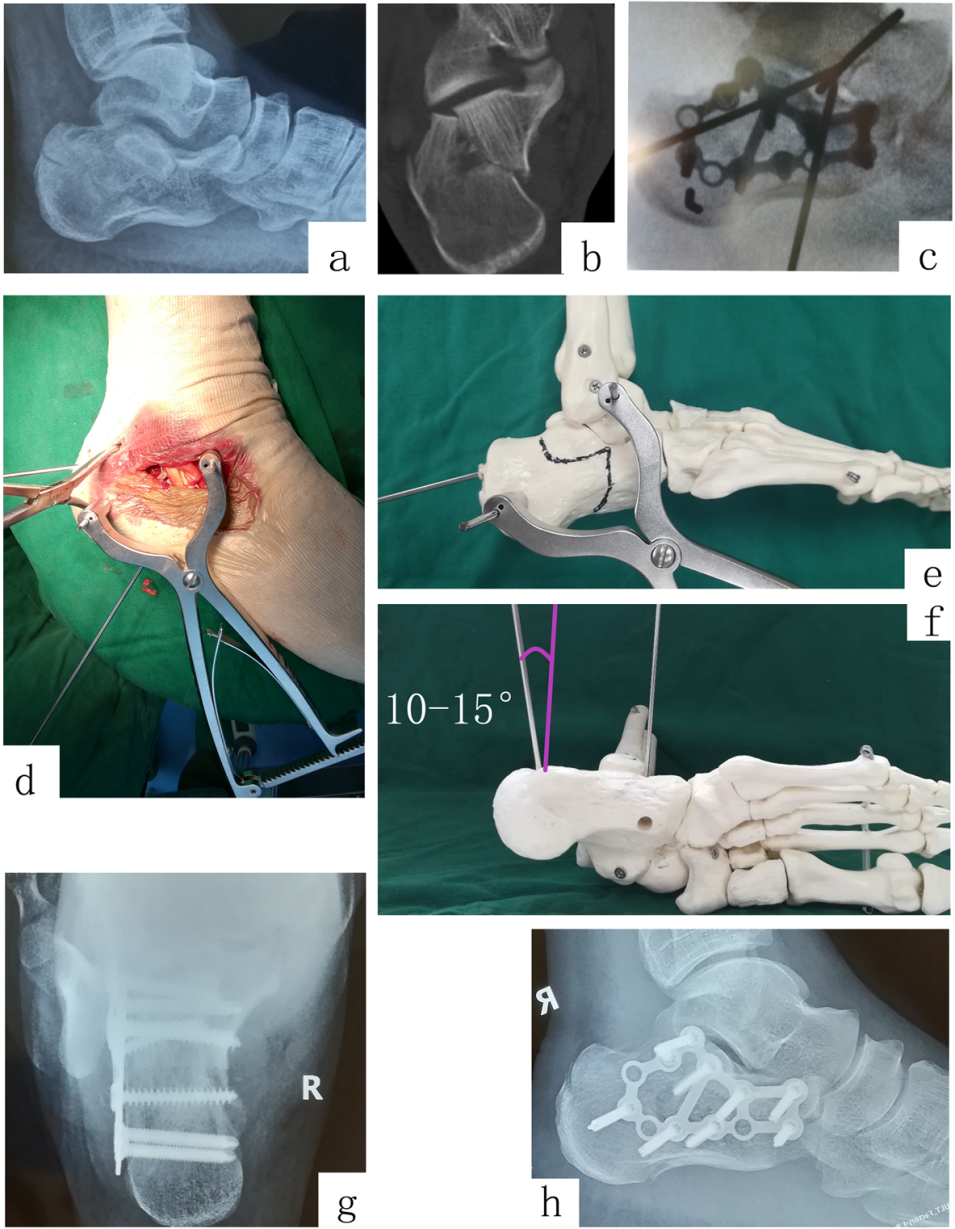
A 27-year-old male patient falls from a height of 2 m. Preoperative lateral (a) and axial (b) radiograph showed left calcaneus fracture with reduced Böhler angle (3°), length (80.1 mm), height (74.7 mm), and width (48.7 mm). The preoperative horizontal computed tomography image showed Sanders type IIB fracture. Steinmann pin retractor-assisted reduction with circle plate fixation via the sinus tarsi approach (c) was used. Postoperative lateral (d) and axial (e) radiographs show obvious correction of the Böhler angle (33°), and improvements in calcaneal length (84.7 mm), height (83.4 mm), and width (40.7 mm). The circle plate was removed (g, f) 17 months post-surgery, and AOFAS score was 87 at the last follow-up (h, i)
